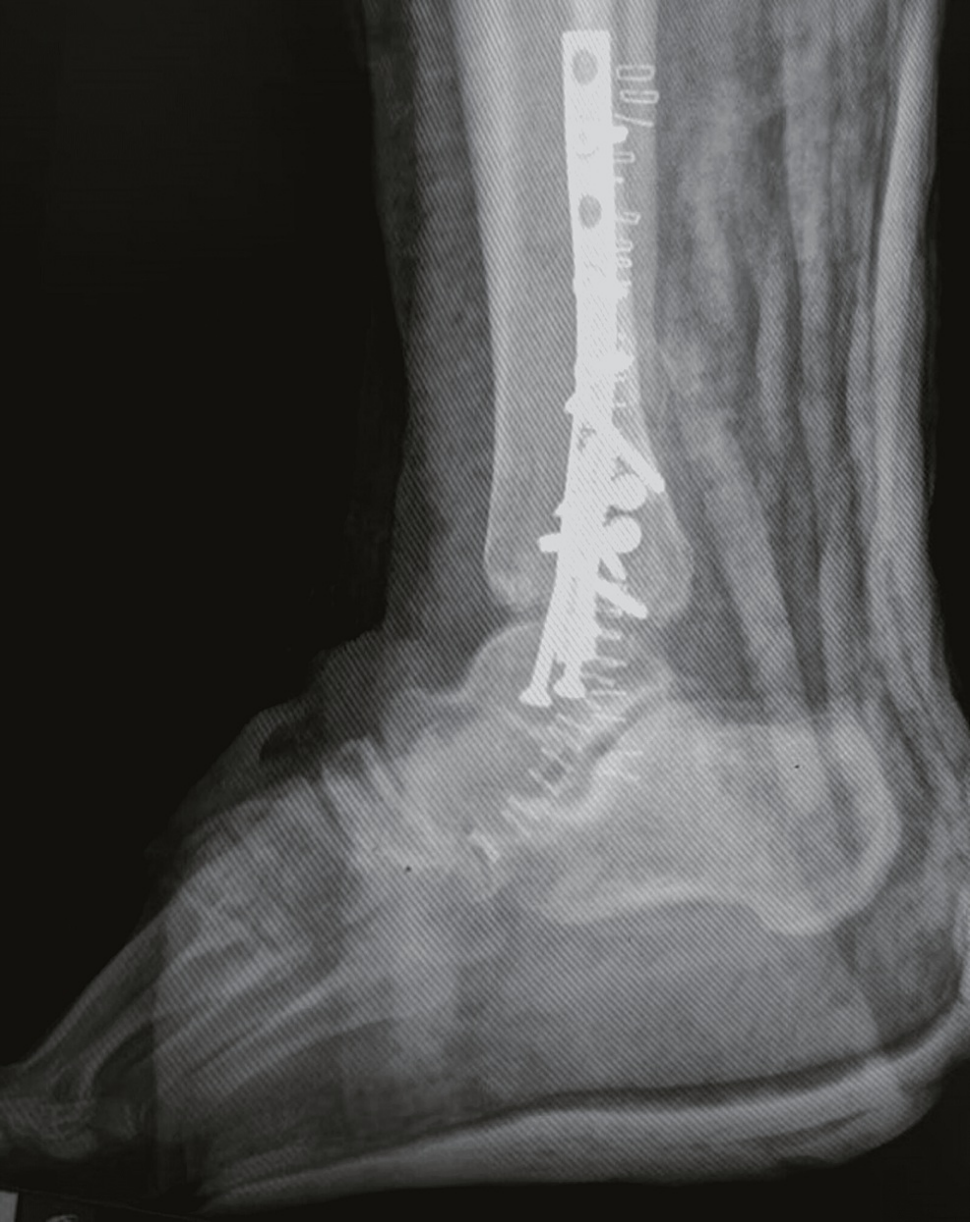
Postoperative radiograph lateral view
